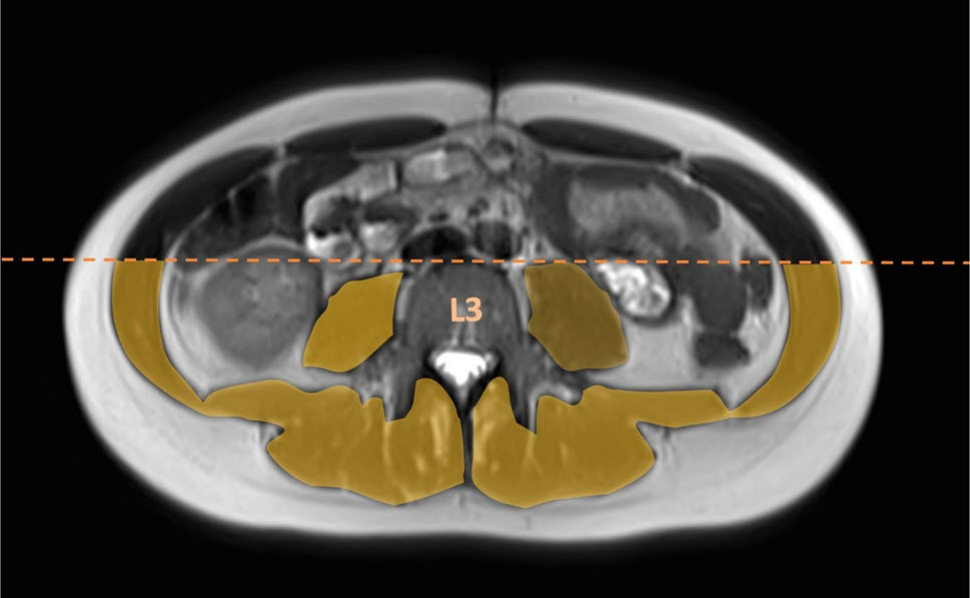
Skeletal muscle area (SMA) on magnetic resonance enterography (MRE). T2-weighted MR images showing the region of interest of dorsal muscles at the level of the third lumbar vertebra (L3)
